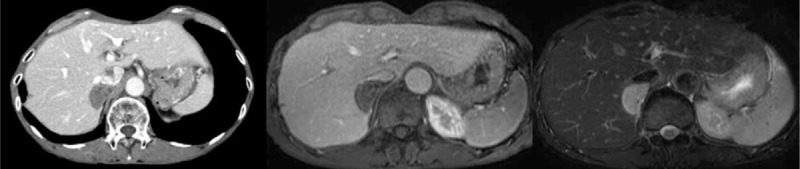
The tumor (approximately 40 mm in diameter) was hypovascular on enhanced computed tomography scan (right), indicated low intensity on T1-weighted MRI (center), and high intensity on T2-weighted or diffusion MRI (left). Dynamic study revealed peripheral enhancement on a late phase. The tumor located close to the inferior vena cava. MRI = magnetic resonance imaging.
